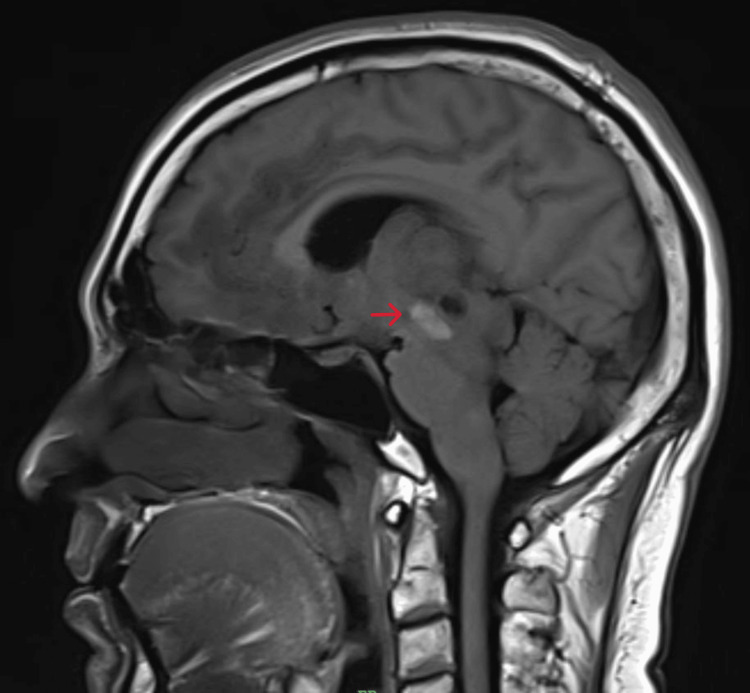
MRI brain T1 - Sagittal section: low T1 signal in infratentorial brain parenchyma
